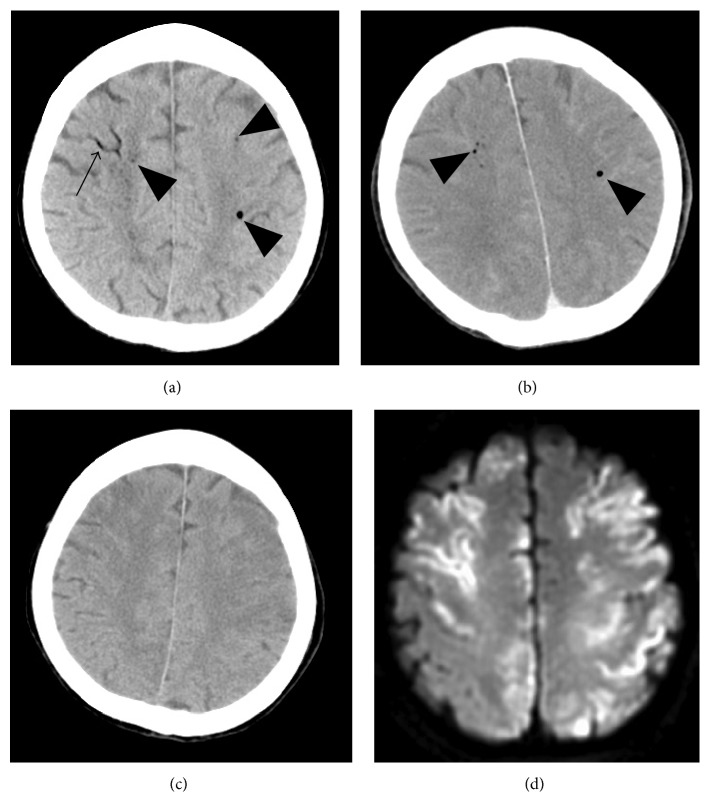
An 82-year-old woman (case 2). (a) Brain CT performed 30 min after notice of her consciousness decrease, that is, 60 min after the estimated onset of CAE, shows air inflow in the cortical veins (arrow) and bilateral cerebral hemispheres (arrow heads). (b) Brain CT obtained 50 min after the first CT scan. The air densities (arrow heads) were diminished. (c) Brain CT obtained 18 hr after the first CT scan. The air densities had completely disappeared. (d) DWI performed one week after onset showing multiple areas of restricted diffusion affecting primarily the cortical areas in the bilateral hemispheres adjacent to air bubbles.
